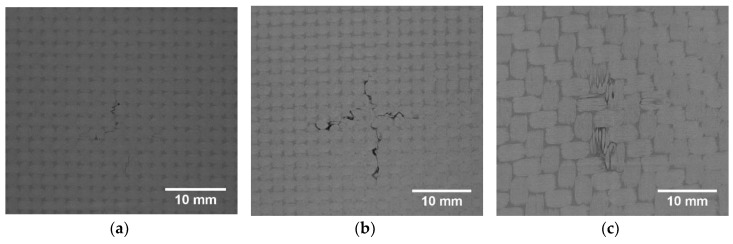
Representative X-ray CT scans with visible damage (from 250 slices): (a) no. 18; (b) no. 20; (c) no. 99.
